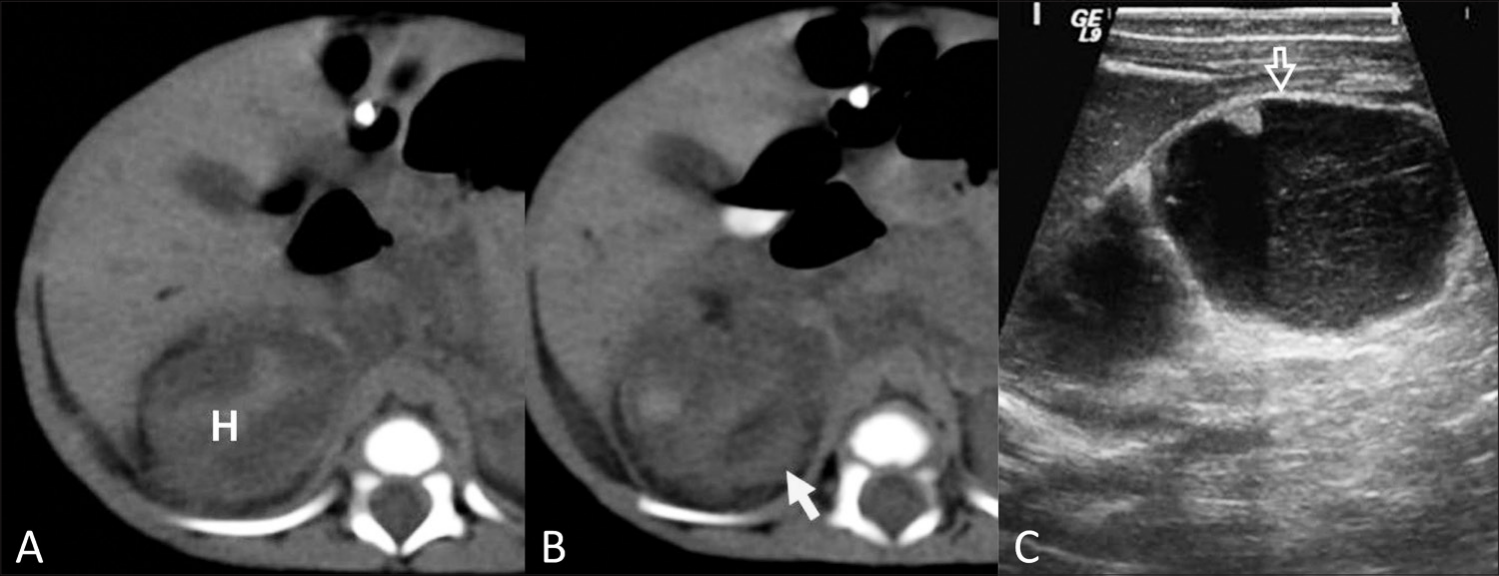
Adrenal hemorrhage: Unenhanced axial CT scans (A,B) at the level of adrenal glands show a heterogeneous mass-like (H) lesion in the right adrenal area with central high density, consistent with blood. The upper pole of the right kidney is seen separately (arrow in B). Follow-up USG for the same patient after 5 weeks shows a complex mass with a mixed, hypoechoic (cystic), and echogenic (solid-like) appearance and peripheral calcific foci (open arrow). The decrease in size suggests resolving hematoma
